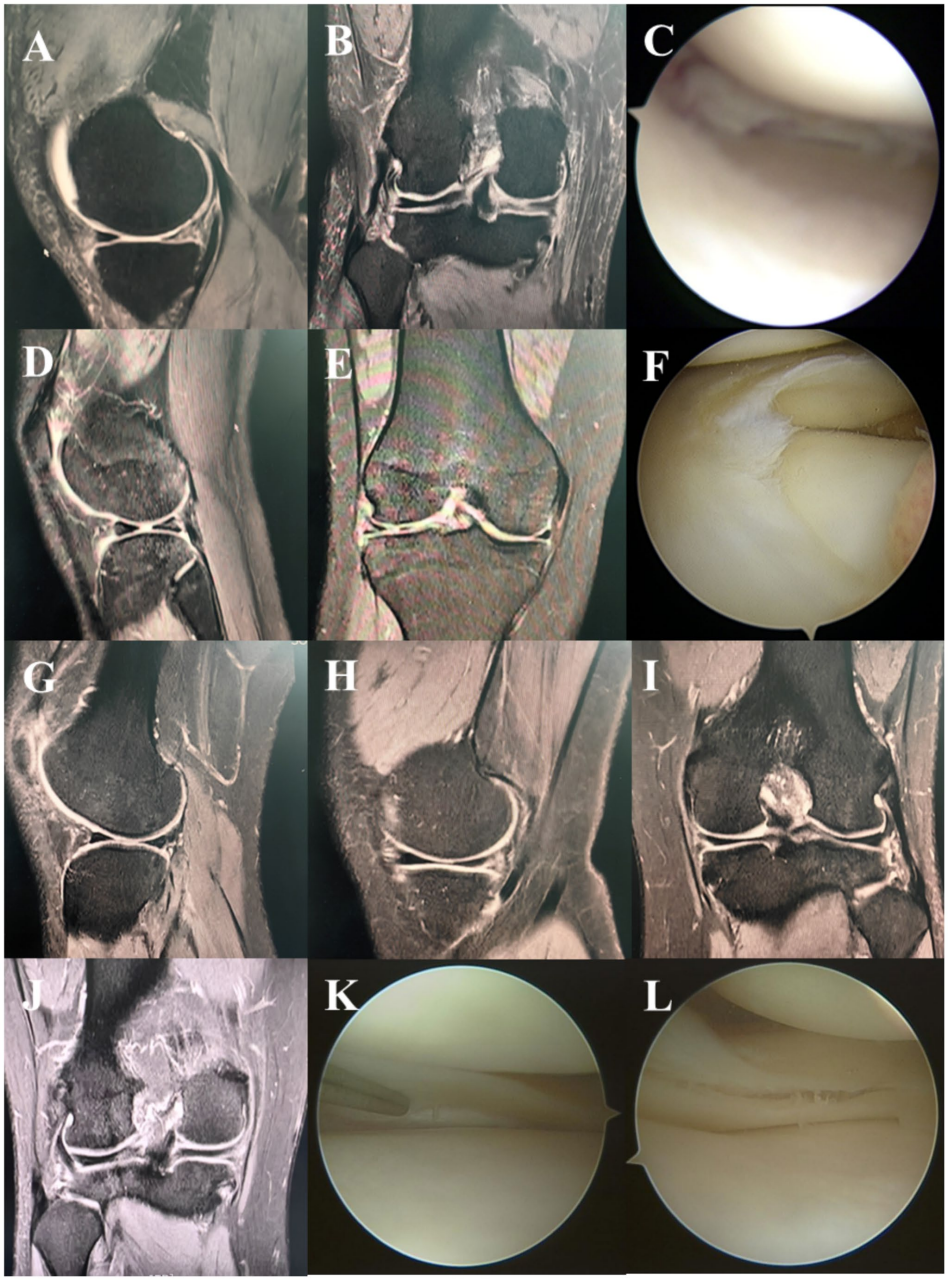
Typical cases. MRI sagittal (A), coronal (B) and arthroscopic (C) manifestations of lateral meniscus injury. MRI sagittal (D), coronal (E) and arthroscopic (F) manifestations of medial meniscus injury. MRI sagittal (G,H), coronal (I,J) and arthroscopic (K,L) manifestations of both lateral and medial meniscus injuries.
